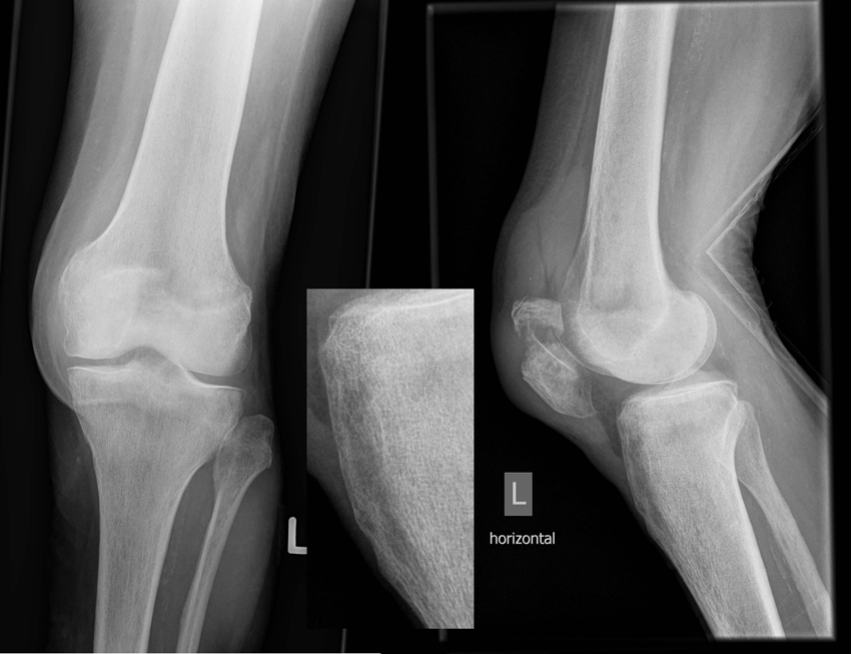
Radiographs directly after fall on ice showing the displaced patellar fracture. Anteroposterior and lateral radiographs showing the transverse displaced patellar fracture and magnified tibial tuberosity indicating no abnormality besides multi-lobular bone-cysts.
